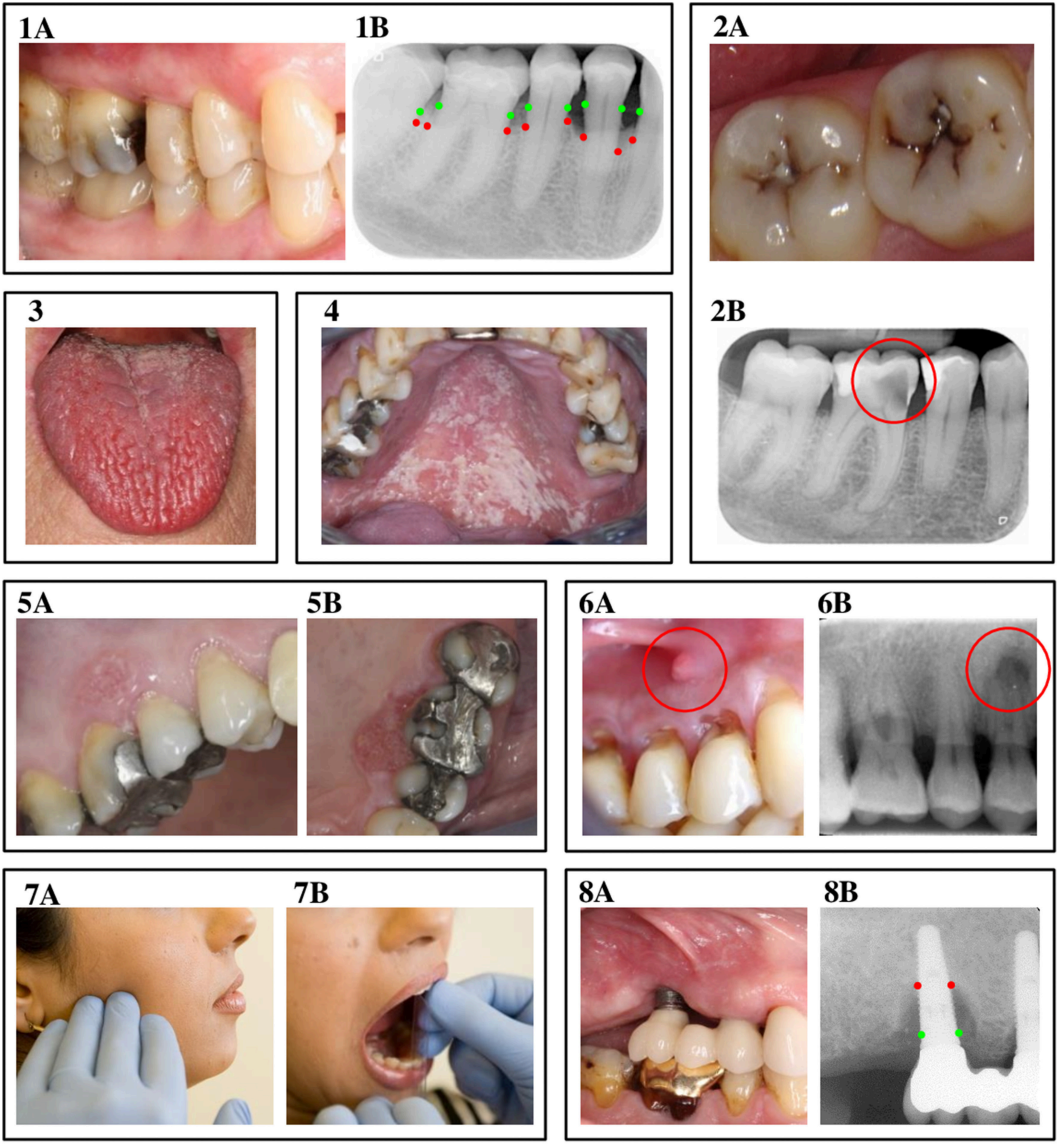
Oral complications of diabetes mellitus. *1A & 1B: Periodontitis*. A clinical view of periodontitis is shown in figure 1A, with the corresponding radiograph displayed in image 1B. The green dots in image 1B indicate where the bone level originally was, while the red dots show the actual bone level as a result of inflammation. *2A & 2B: Dental caries*. Image 2A displays a clinical view of dental caries, observed from the occlusal view. Picture 2B is a radiograph of an example of a deep carious lesion, marked by the red circle. *3. Hyposalivation*. The photo in image 3 shows a clinical view of fissured tongue, caused by severe hyposalivation. *4. Oral candidiasis*. Image 4 displays a clinical view of oral candidiasis, located at the palate. *5A & 5B: Oral cancer*. At image 5, a clinical representation of leukoplakia and oral cancer is shown at the buccal (5A) and palatal (5B) site. *6A & 6B: Apical periodontitis*. Figure 6A shows a fistula, caused by apical periodontitis, with image 6B as the corresponding radiograph, where the lesion at the apex of the tooth is marked by the red circle. *7A & 7B: Temporomandibular disorders*. Image 7A indicates the location of pain often seen in patients with temporomandibular disorders, while 7B shows a measurement of limited jaw opening, another symptom of temporomandibular disorders. *8A & 8B: Peri-implantitis*. At figure 8A, a clinical view of peri-implantitis is presented, with 8B as the corresponding radiograph. Again, the red dots indicate where ideally, the bone level should be, while the red dots show the actual reduced bone level as a result of the inflammatory process. The authors thank the following colleagues for providing clinical pictures and/or radiographs: Dr. AJP van Strijp (dental caries), Prof. Dr. M Laine (hyposalivation), Dr. P Wetselaar (temporomandibular disorders and apical periodontitis), all from ACTA, the Netherlands; Dr. RJJ van Es (oral cancer), UMC Utrecht, The Netherlands; Prof. Em. Dr. I van der Waal (oral candidiasis).
